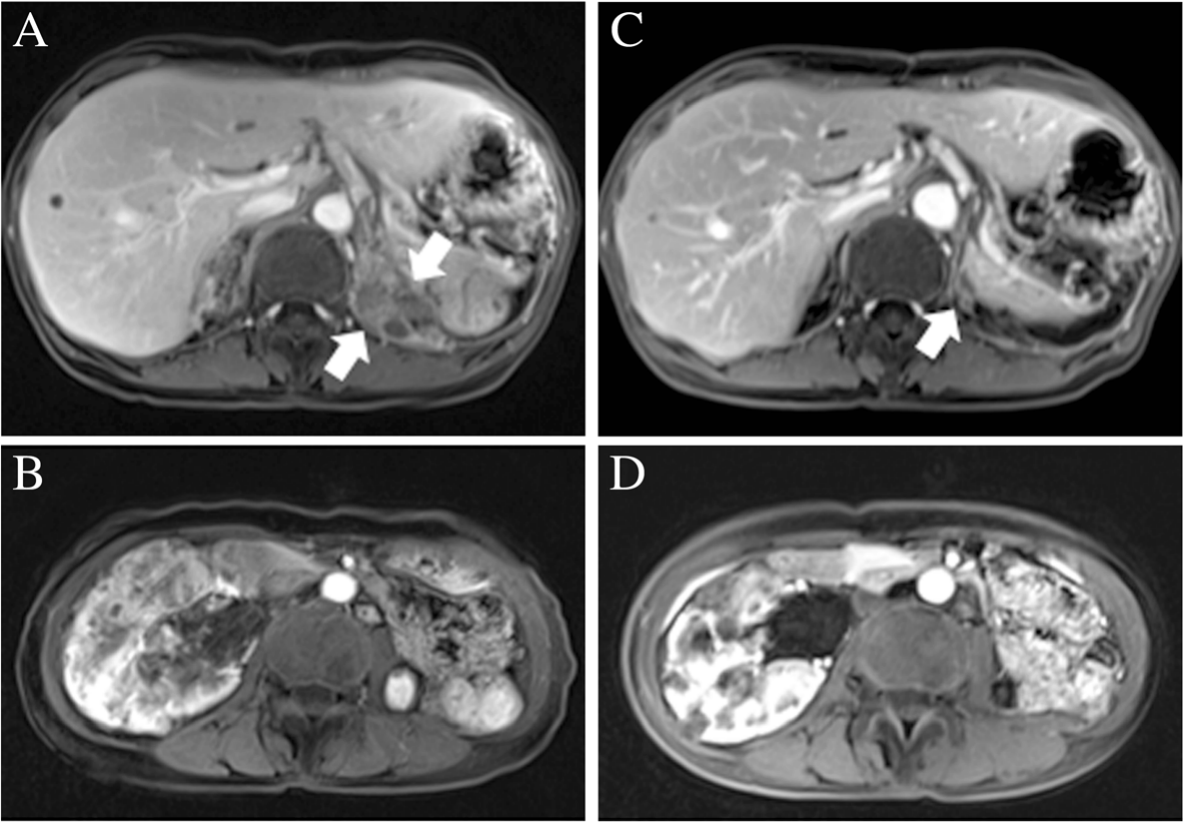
Magnetic resonance images showing the treatment response of recurrent renal cell carcinoma and angiomyolipoma. (A) Magnetic resonance imaging was used to produce this image showing recurrent renal cell carcinoma at the posterior aspect of left upper quadrant of abdomen (arrows). (B) Magnetic resonance imaging scan showing angiomyolipoma. (C) Magnetic resonance imaging scan showing partial response to everolimus treatment of renal cell carcinoma close to left diaphragm (arrow). (D) Imaging study showing regression of the patient’s angiomyolipoma.
